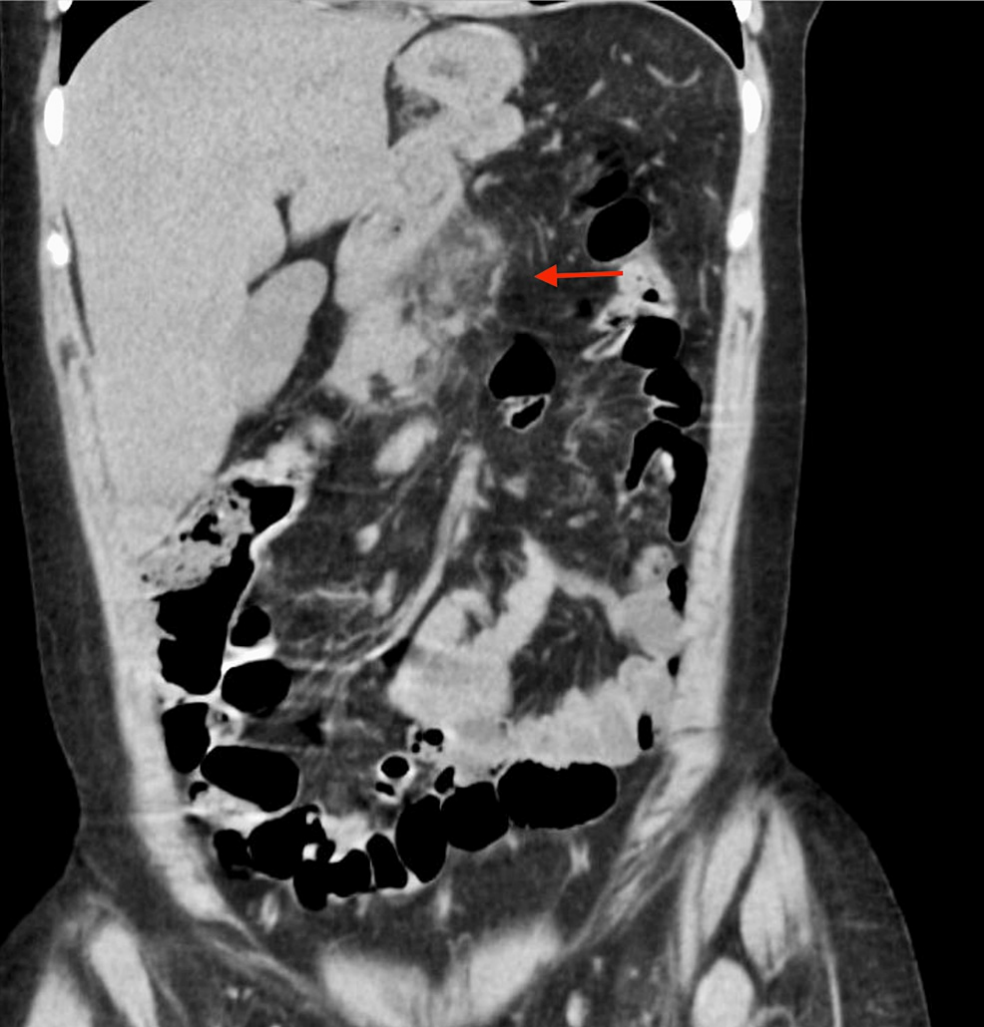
Vertical cross-section CT imaging of the abdomen with the arrow depicting edema surrounding the pancreas.
